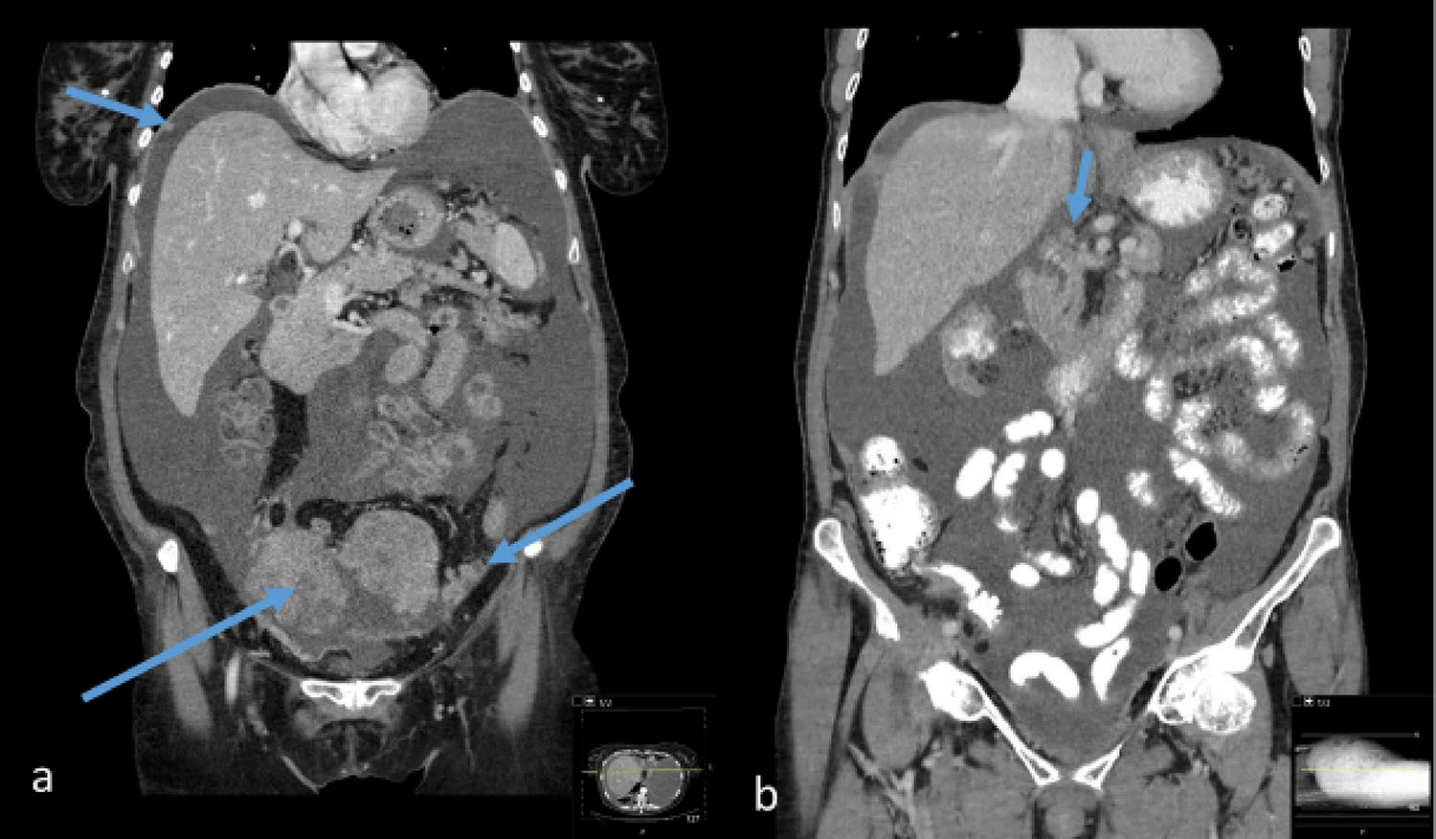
Peritoneal carcinomatosis and ascites in patients with ovarian cancer. a CT-image (contrast-enhanced CT of the abdomen and pelvis, coronary projection) showing right diaphragmatic carcinomatosis, left-side pervic carcinomatosis, and pelvic masses. b CT-image (intravenous and oral-enhanced CT of the abdomen and pelvis, coronary projection) carcinomatosis in truncus coeliacus area
